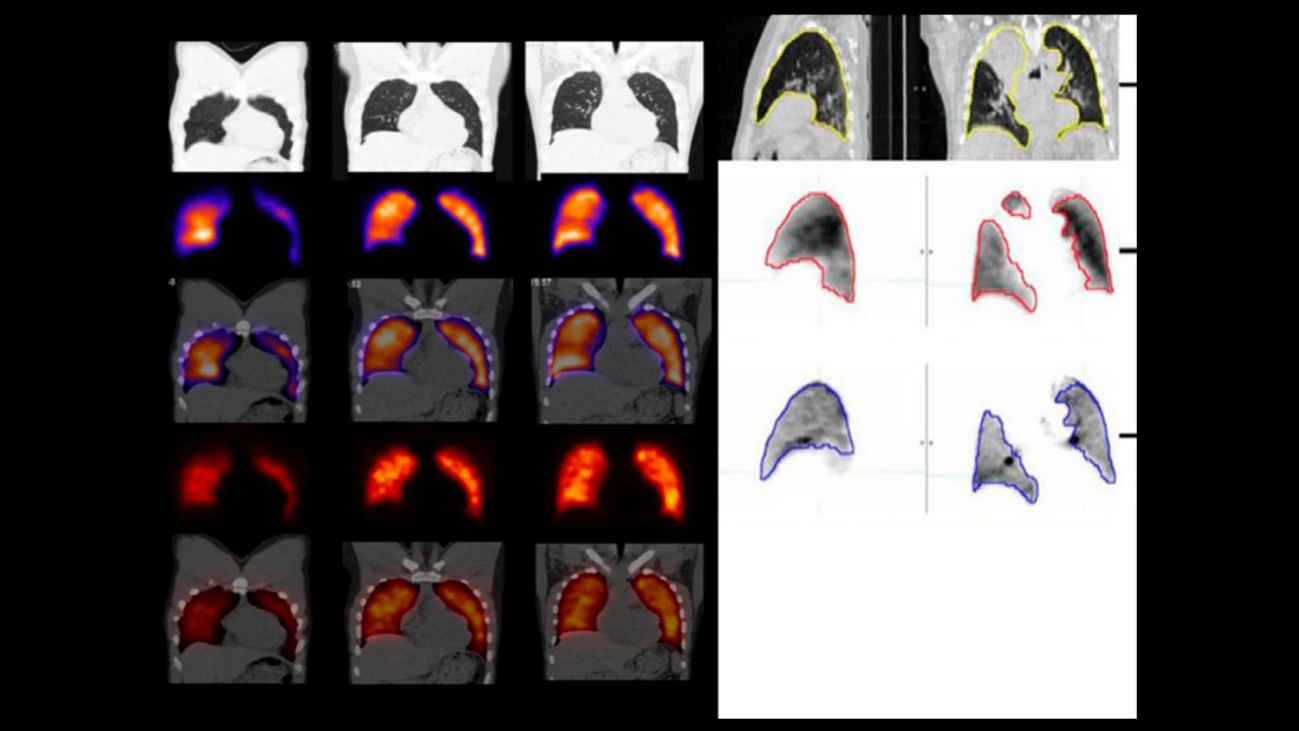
À esquerda, série de imagens exemplificando uma cintilografia pulmonar V/Q Spect-CT normal, com uso de technegas/MAA marcados com 99mTc, mostrando de cima para baixo: TC baixa dose; ventilação Spect; fusão da ventilação/TC; perfusão Spect; e fusão perfusão/TC. À direita, série de imagens exemplificando um V/Q PET-CT normal, com uso de Galligas/MAA marcados com 68Ga de cima para baixo, cortes sagitais de fusão da ventilação/TC (esquerda) e perfusão/TC (direita). Adaptado de Le Roux Pyet et al.279e Bailey DL et al.
